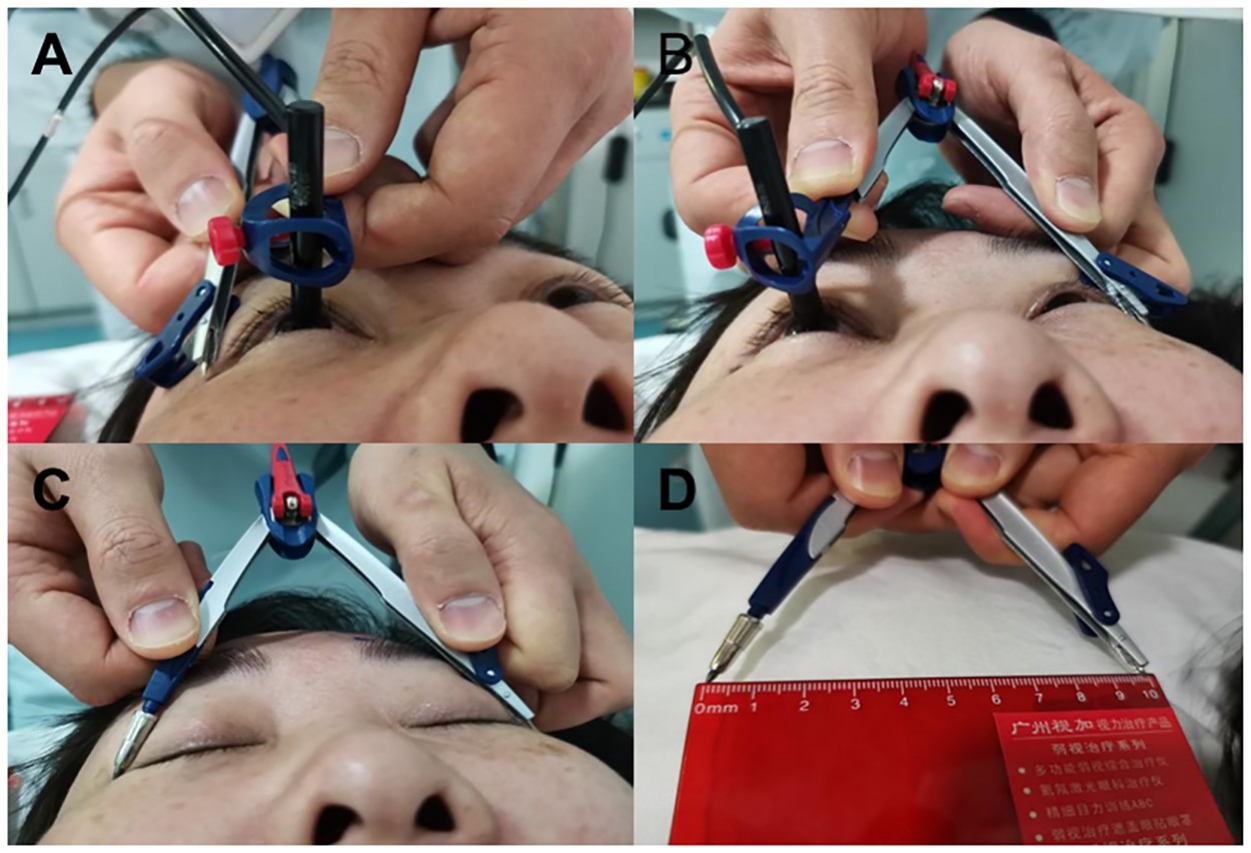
Using the Amplitude-mode Ultrasound probe and compasses to measure the distance from corneal apex to bilateral orbital margin (A and B). Measuring the transverse distance between the bilateral orbital margin marker (C). The distance was measured with a ruler (D).
